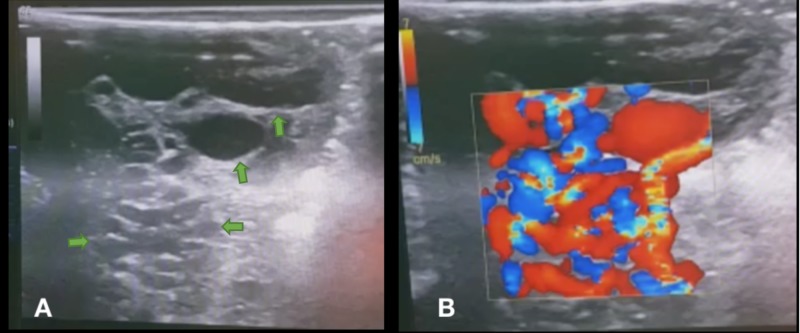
Intraoperative ultrasound A: Gray-scale ultrasound with linear probe showing multiple hypoechoic/anechoic tubular structures (arrows) within the brain parenchyma. B: Doppler function showing bidirectional, high-blood flow within the tubular structures, suggestive of arteriovenous malformation (AVM) vessels.
